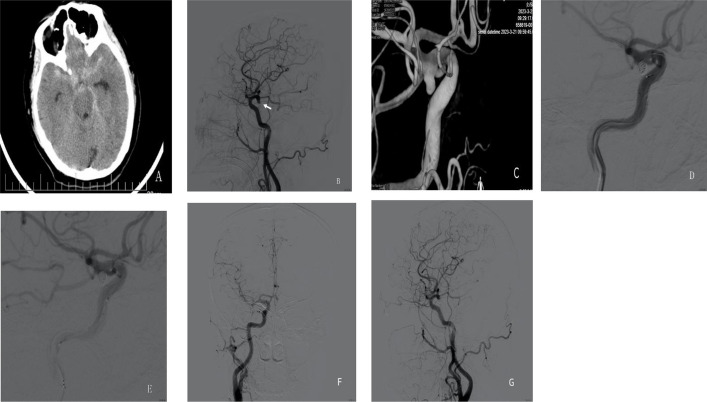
Treatment of the right posterior communicating artery aneurysm with LVIS stent-assisted coiling. A Admission non-contrast axial cranial CT demonstrated extensive subarachnoid hemorrhage in the basal cisterns and sylvian fissures (arrowheads), consistent with acute aneurysmal rupture. B Right internal carotid artery angiogram (lateral view) revealed a saccular aneurysm (arrow) originating from the posterior communicating artery. C Three-dimensional reconstructed angiographic image provided detailed visualization of the aneurysm morphology, neck configuration (asterisk), and its spatial relationship to the parent artery. D, E Immediate postprocedural angiograms obtained in working projections demonstrated complete aneurysm occlusion according to the Raymond–Roy Occlusion Classification (RROC class I) without residual contrast filling. The deployed LVIS stent adequately covered the aneurysm neck (arrowheads) with preserved patency of the parent vessel. F, G Six-month follow-up angiograms in anteroposterior (F) and lateral (G) views confirmed maintained complete occlusion (RROC class I) without evidence of aneurysm recurrence or in-stent stenosis
